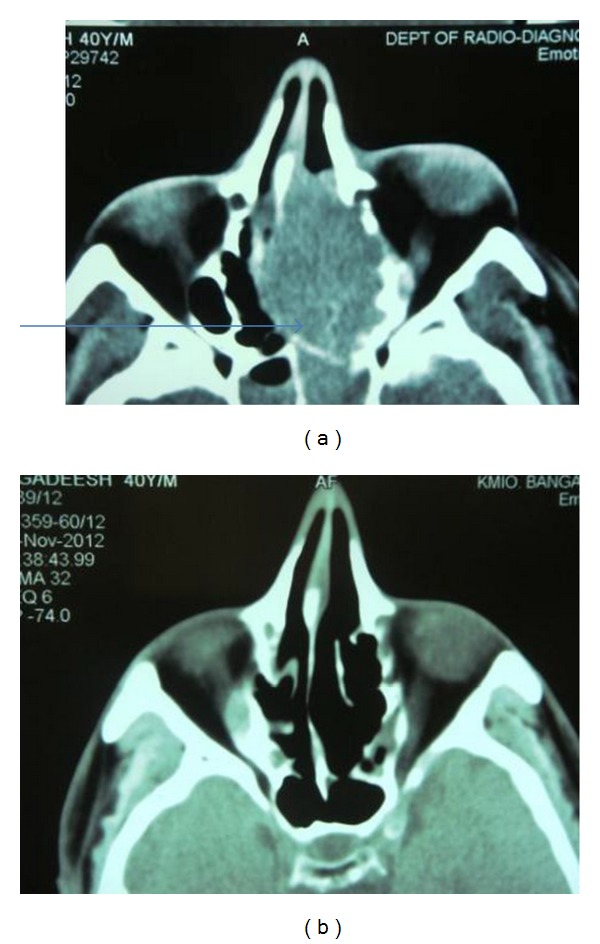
Computed tomography (CT) of paranasal sinuses and orbit showing mass (arrow) occupying the entire left ethmoid and sphenoid sinus extending into left nasal cavity causing erosion of nasal septum, extending into orbit and intracranial extension into basifrontal area (before chemoradiation and after chemoradiation).
